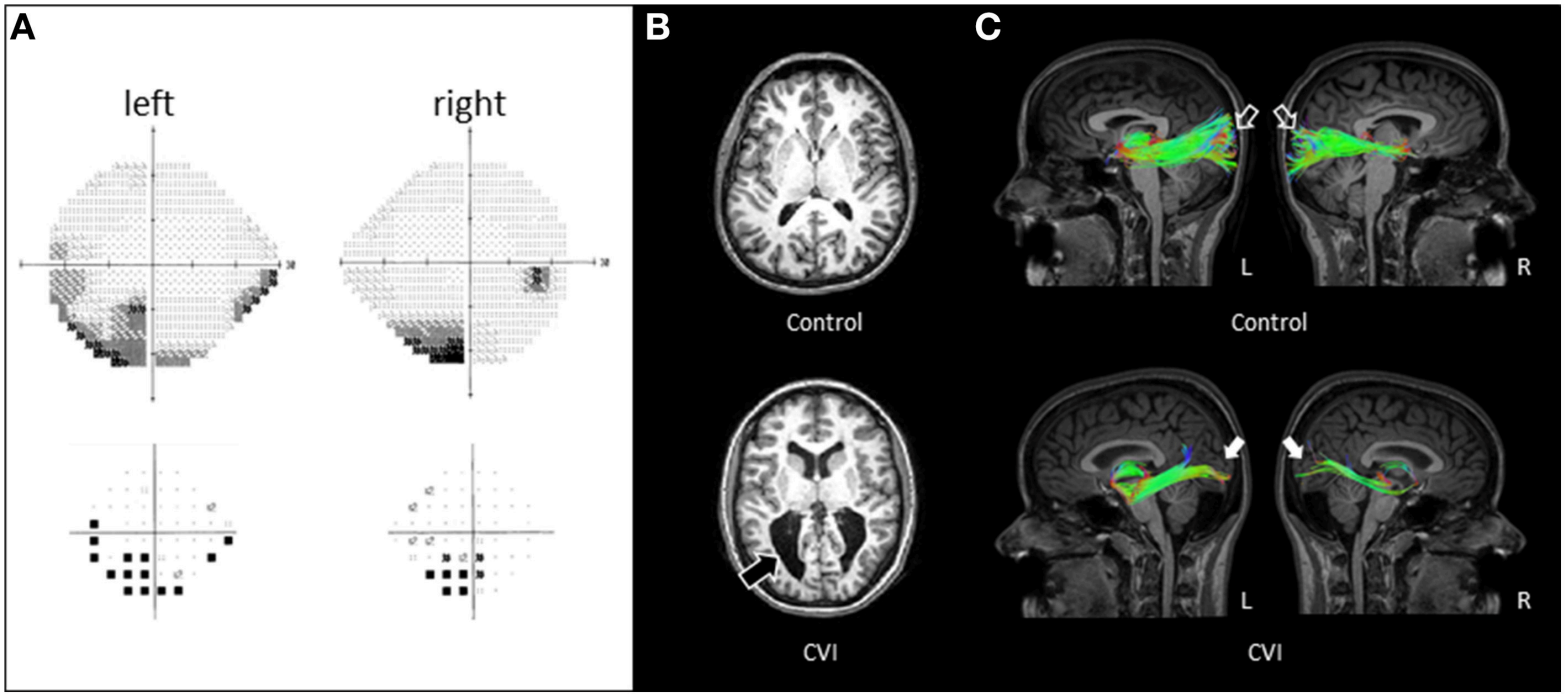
(A) Visual field assessment of CVI patient obtained by Humphrey automated visual perimetry (see text for test details). A bilateral field defect involving the inferior visual fields (with a more dense defect involving the left side) in each eye evident on the gray scale plot (top panel) and confirmed on the pattern deviation plot (bottom panel). (B) Axial T_1_-weighted MRI images (MP-RAGE pulse sequence) in a normally sighted control (top panel) and CVI patient (lower panel). Enlarged lateral ventricles with irregular posterior borders are apparent in the CVI patient (black arrow). (C) Corresponding white matter tractography of the optic radiations (sagittal view) revealed with HARDI in the same individuals. Note in the control subject, the complete arborization of the superior and inferior banks of the optic radiations extending from the thalamus to the occipital cortex in both hemispheres (open arrows). In contrast, the CVI patient shows markedly fewer projections and in particular, along the superior bank of the optic radiations (white arrows). The paucity of connections along the superior bank along with a greater deficit of connections in the right (R) compared to the left (L) hemisphere correspond to the location of the visual field deficit of the CVI patient characterized by automated perimetry.
